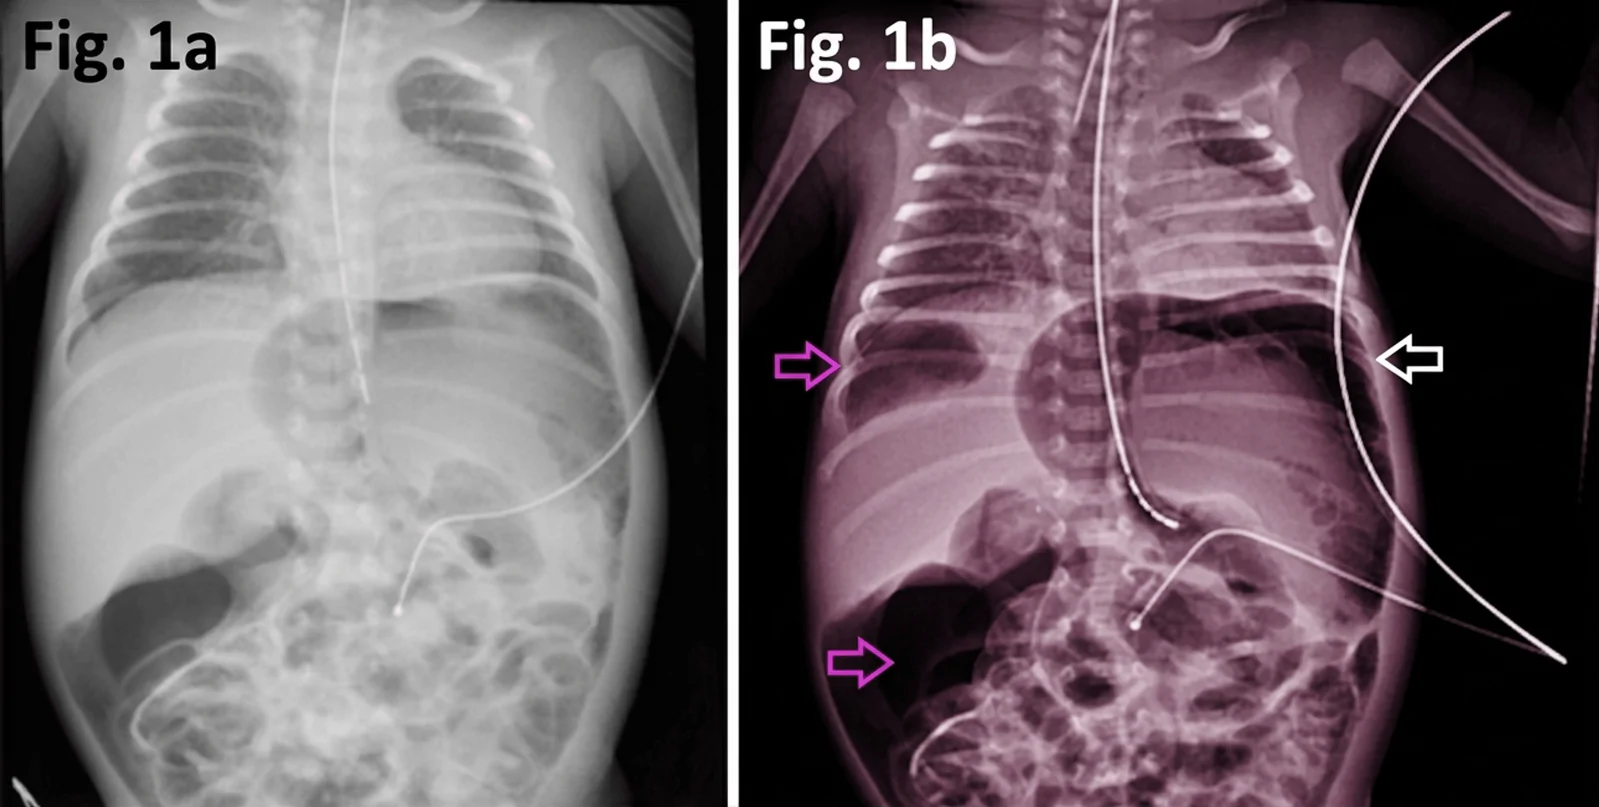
An abdominal X-ray showing sudden recurrence of significant pneumoperitoneum. (a) Description: anterior-posterior (AP) supine X-ray of the chest and abdomen of an infant. Shows various support tubes in situ and a general overview of the anatomy. A small gas bubble is seen under the left diaphragm, which is common and often benign (gastric bubble), contrasting with the diffuse appearance of pathology.
